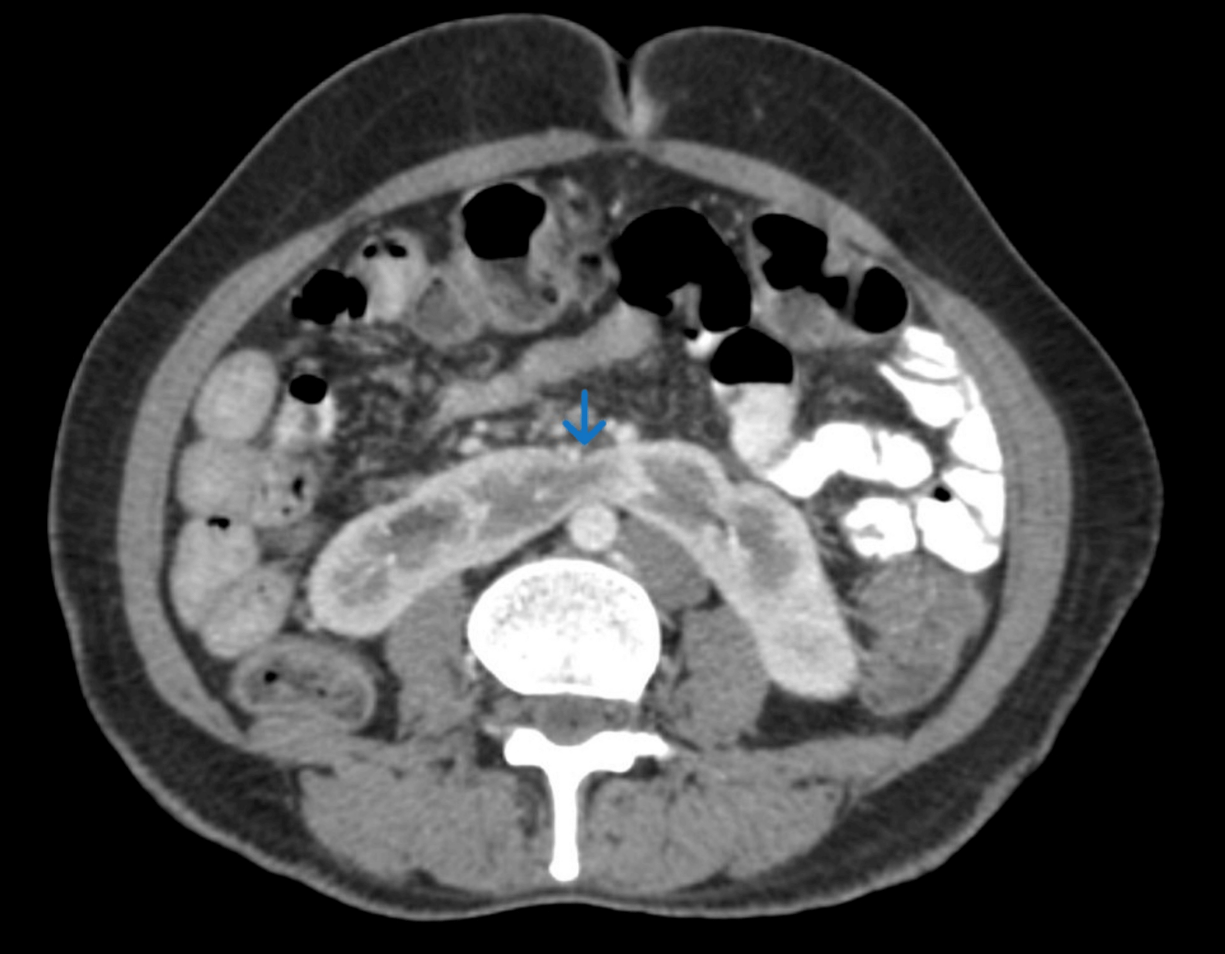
Axial late arterial phase contrast CT scan of the abdomen shows the horseshoe kidney CT: computed tomography
